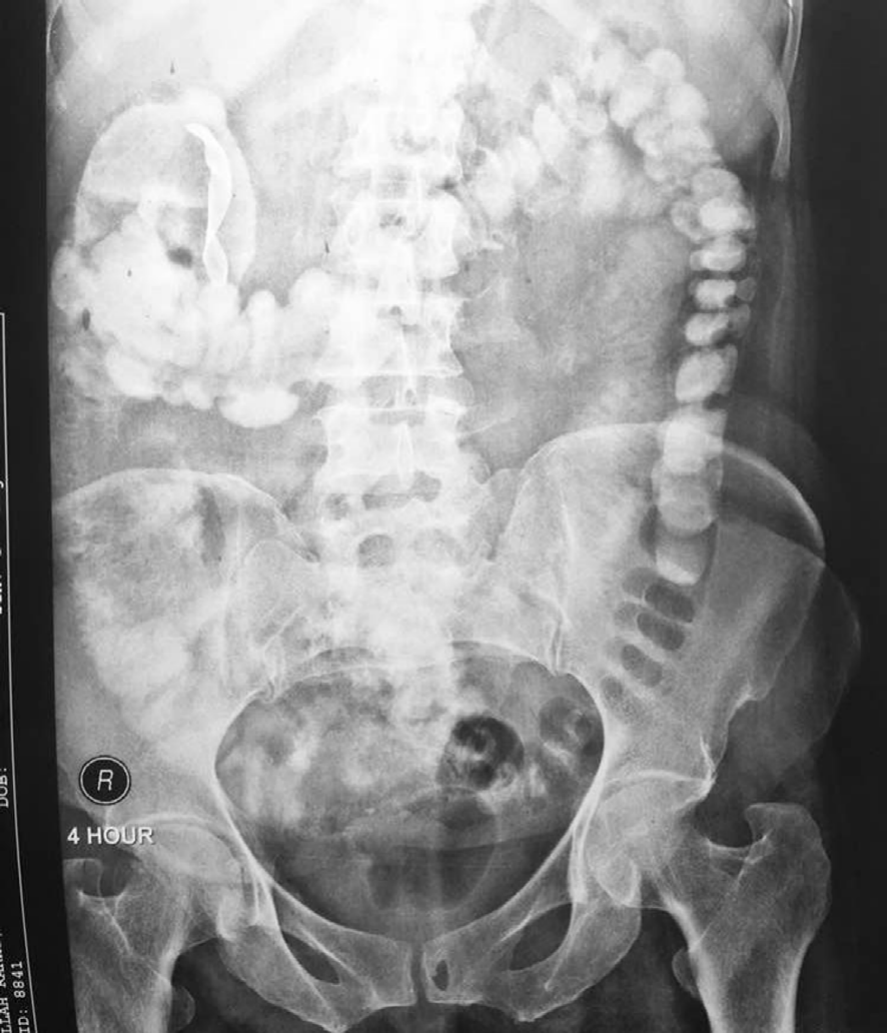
X-ray at 4 hour showing gauze in ascending colon.
